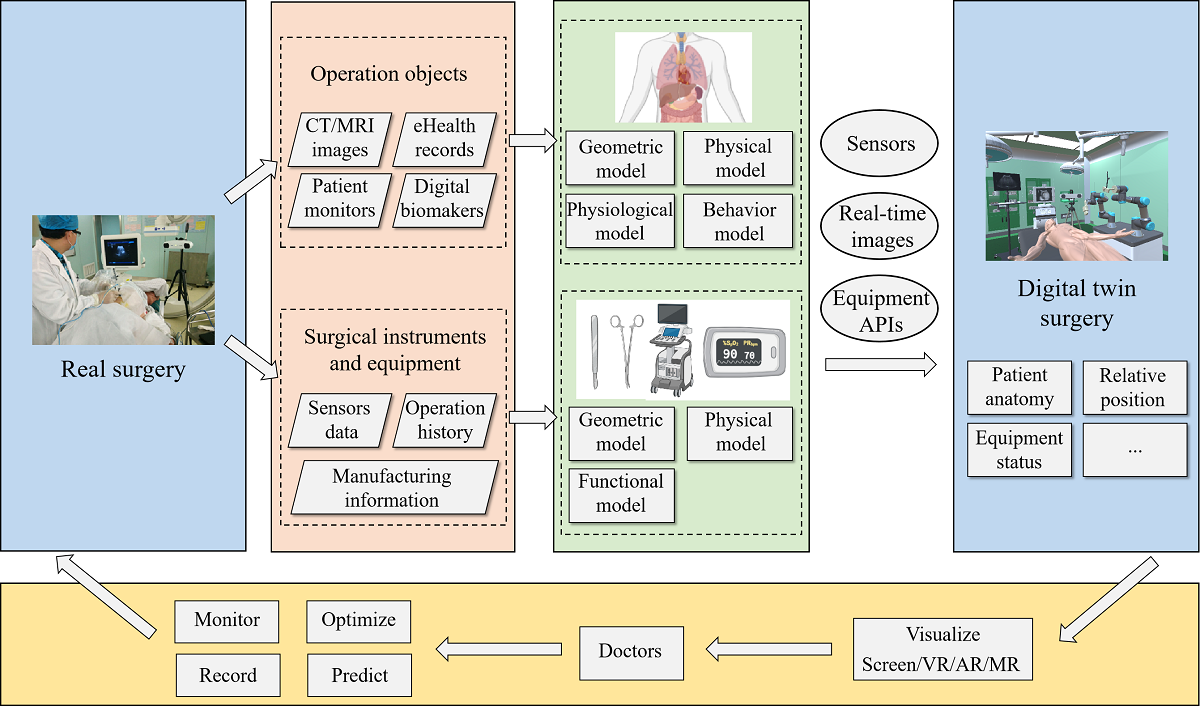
Digital twin–based computer-assisted surgery (CAS) solution. With the support of digital twin surgery, we can perform monitoring, optimization, recording, and prediction for the real surgery. API: application programming interface; AR: augmented reality; CT: computed tomography; MR: mixed reality; MRI: magnetic resonance imaging; VR: virtual reality.
